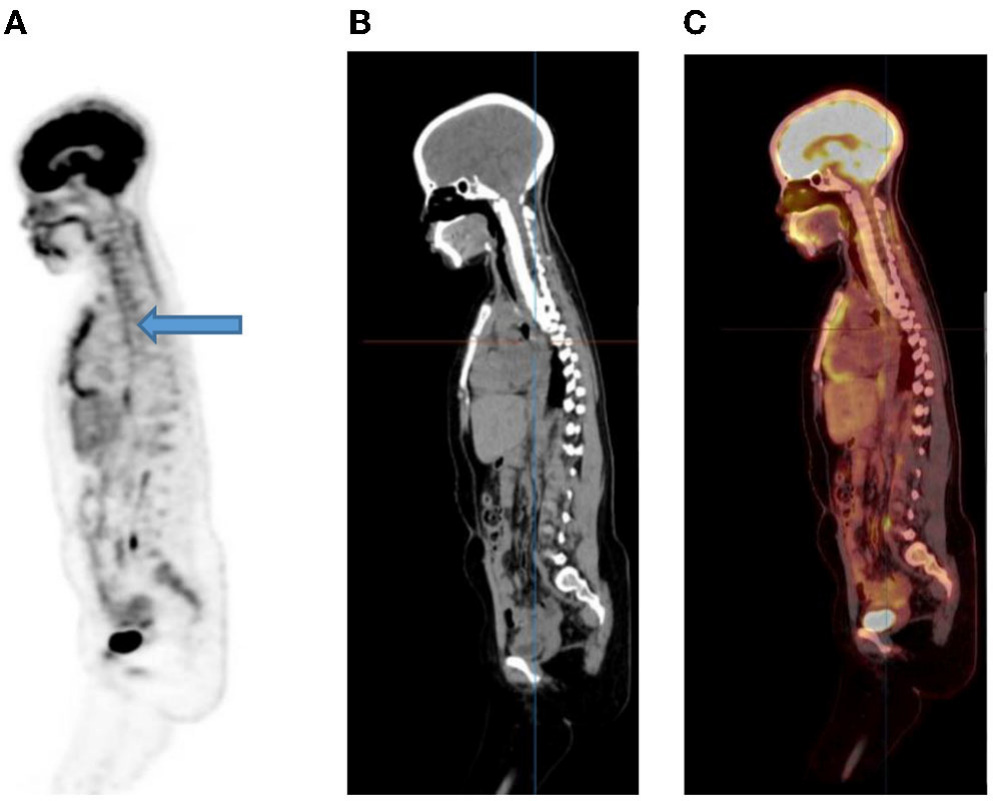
A 15-year-old male known with Hodgkin's lymphoma, referred for a restaging PET/CT post chemotherapy. Sagittal PET (A) showing linear uptake anterior to the thoracic spine corresponding to uptake in the esophagus (blue arrow), likely attributed to reflux or esophagitis. The CT (B) and fused PET/CT (C) will assist in this interpretation too.
